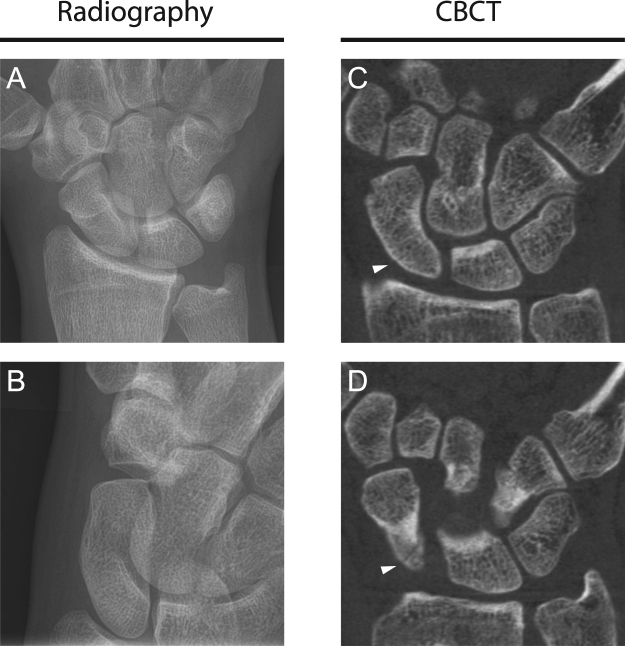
29-year-old male patient who suffered from trauma presented in the ED. Radiographs and CBCT were acquired because scaphoid fracture was suspected. No fracture is seen in the radiographs (A dorsopalmar, B dorsopalmar with ulnar deviation) whereas the coronal reconstructions of the CBCT (C,D) clearly depict the fracture (white arrowhead).
